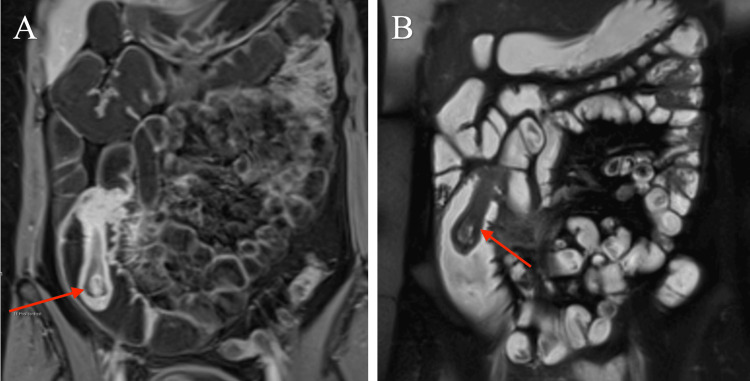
(A) MRI abdomen after contrast showing enhancing polypoidal mass at the terminal ileum (red arrow). (B) Coronal T2 MRI showing dilated terminal ileum and the polyp (red arrow)
